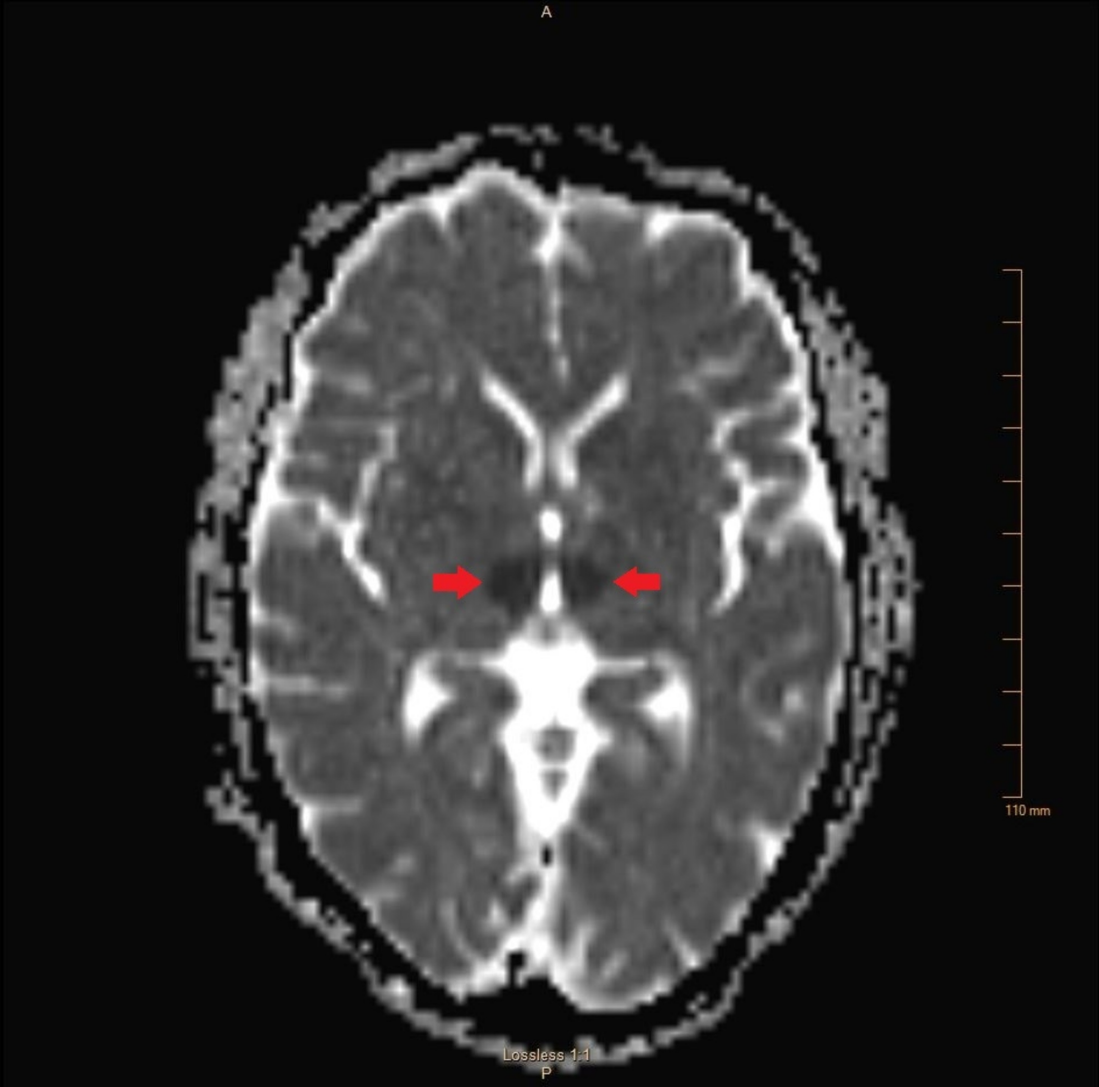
MRI with apparent diffusion coefficient confirming infarct in the area supplied by the AOP. MRI, magnetic resonance imaging; AOP, artery of Percheron.
